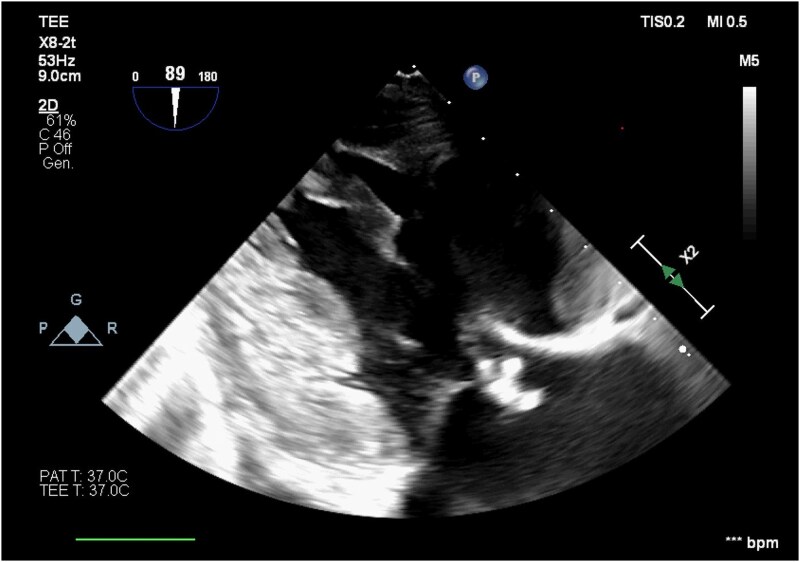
TOE in *trans*-gastric view showing a mass attached to the prolapsing leaflet corresponding to postero-medial PM head detachment. TOE, transoesophageal echo, PM, papillary muscle.
